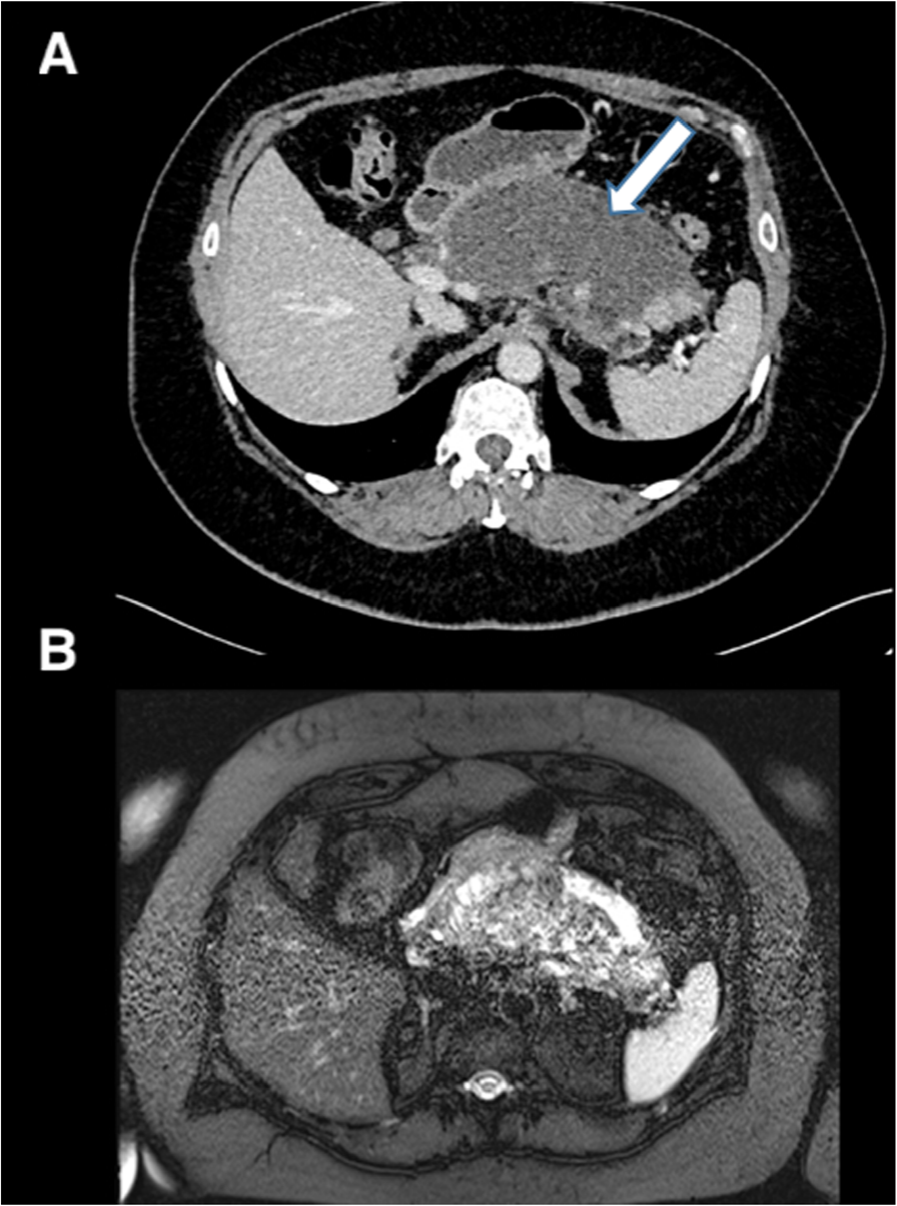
Axial contrast-enhanced CT of the abdomen (a) and axial fat-suppressed T2-weighted MRI of the abdomen (b) in the same patient demonstrating extensive inflammation and oedema of the pancreas secondary to gallstone pancreatitis with a peripancreatic collection (arrow)
